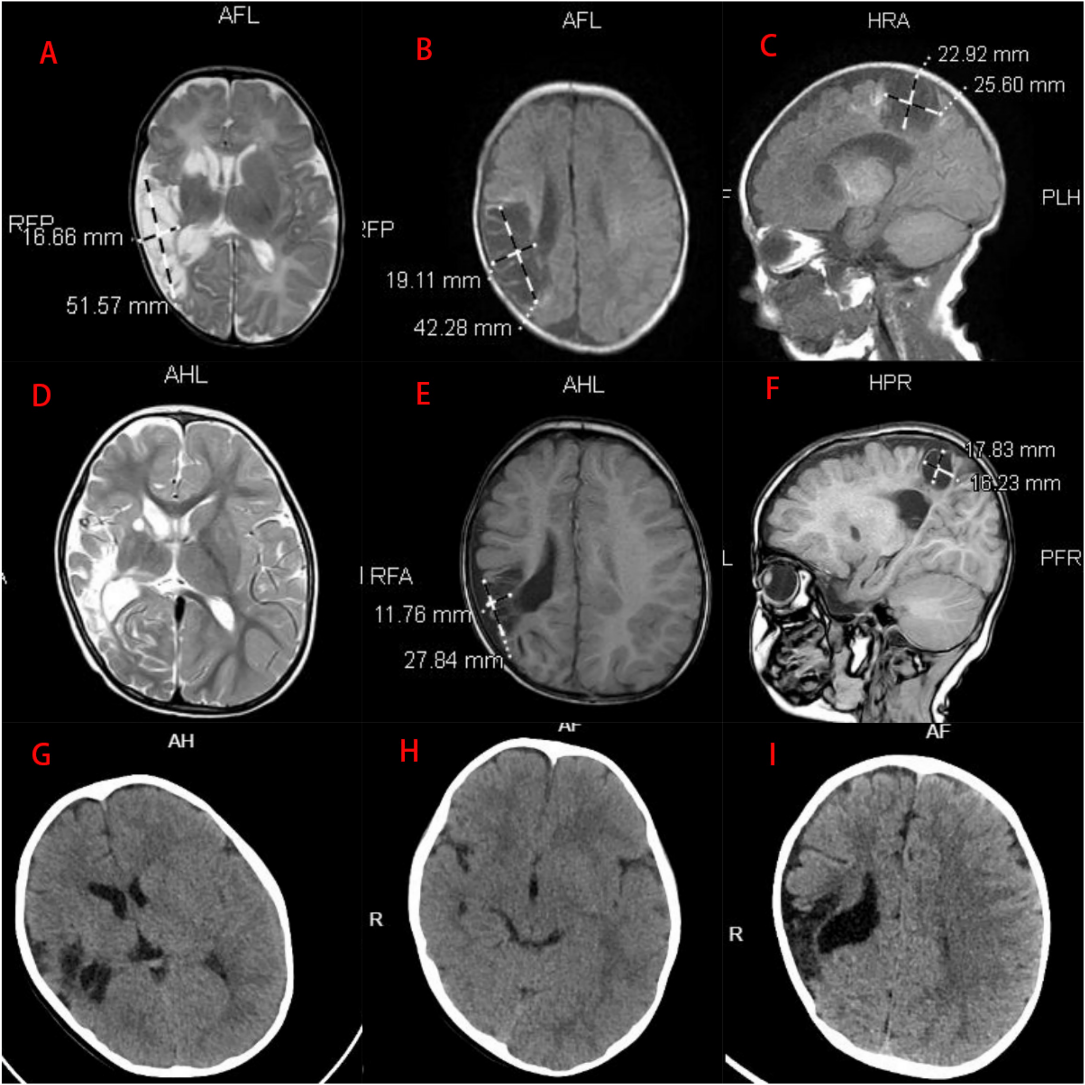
(A–C) Brain MRI at day 40 of life showed extensive encephalomalacia localized to the right fronto-temporal-parietal lobe and right basal ganglia (same scale). (D–F) Follow-up MRI at month 18 demonstrated stabilization of encephalomalacia in these regions (same scale). (G–I) Brain CT at month 24 revealed right hemispheric volume reduction with hypodense lesions in the right temporoparietal areas.
